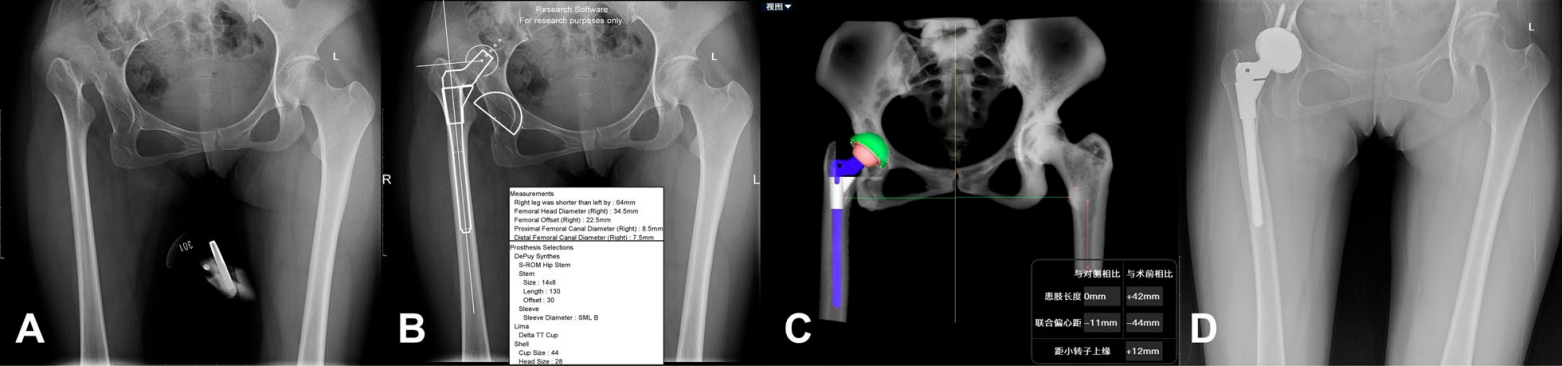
Case 4, woman, 35 years old, Crowe IV DDH, right. A Standard preoperative anteroposterior X-ray of the pelvis. B Preoperative planning by the 2D template; planning results: Cup size was 44 mm (Since the OrthoView software does not have a 44 mm Pinnacle cup, a 44 mm Lima cup was planned by the 2D template preoperatively, but the Pinnacle cup was used intraoperatively. C Preoperative planning by AIHIP; planning results: Cup size was 44 mm. D Standard postoperative anteroposterior X-ray of the pelvis: Cup size was 44 mm
